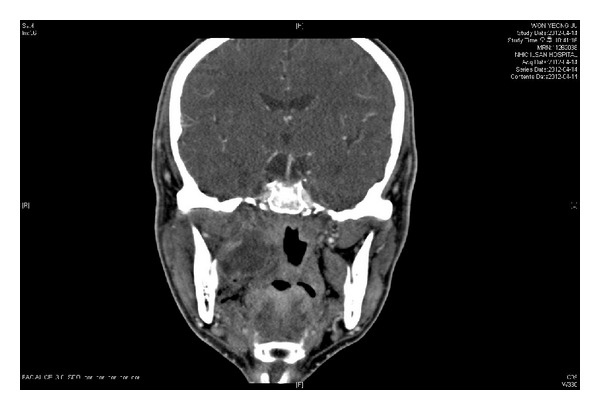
On CT images, an abscess pocket had diffused from the right pterygomandibular area to the right cavernous sinus area.
